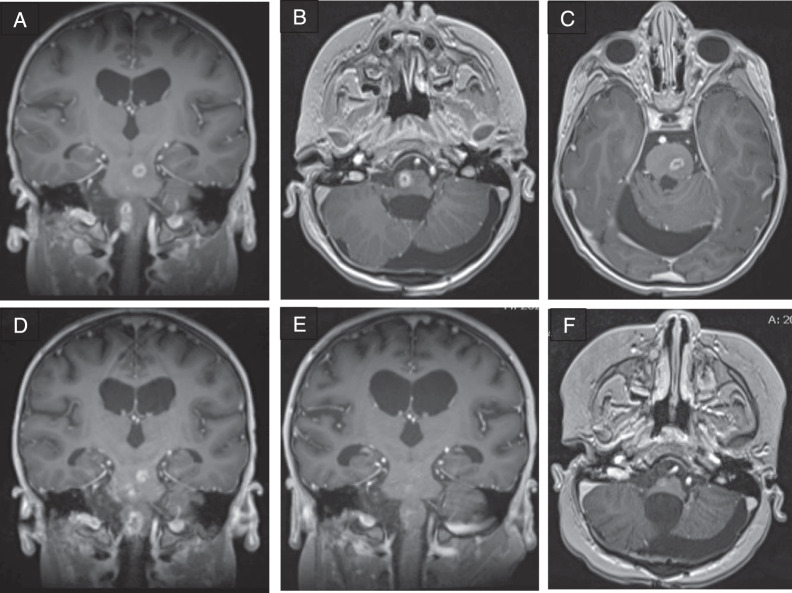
Development of rim enhancing lesions within the brainstem 5 months after radiation therapy. (A–C) Postgadolinium T1-weighted imaging approximately 5 months after radiation. (D) Postgadolinium T1-weighted imaging 6 weeks after initial imaging. (E and F) Postgadolinium T1-weighted imaging after completion of bevacizumab therapy.
